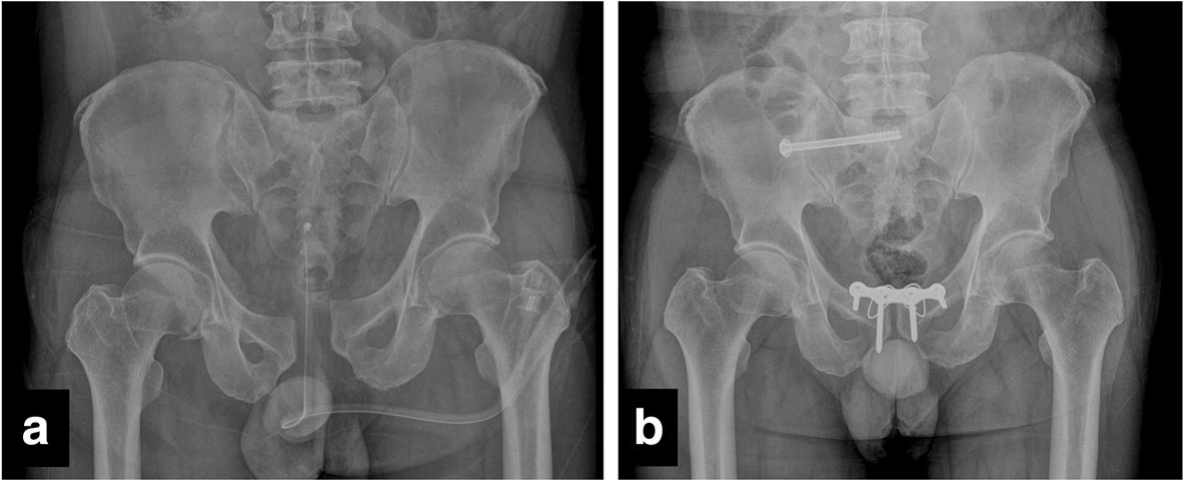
Plain pelvis radiograph. a Preoperative image of a 36-year-old male patient after pelvic ring injury (61-B1). b A follow-up image taken 1 year after surgery, showing anterior fixation with plate and screw fixation with tension band wiring and posterior fixation with an iliosacral screw for a right sacroiliac joint disruption
